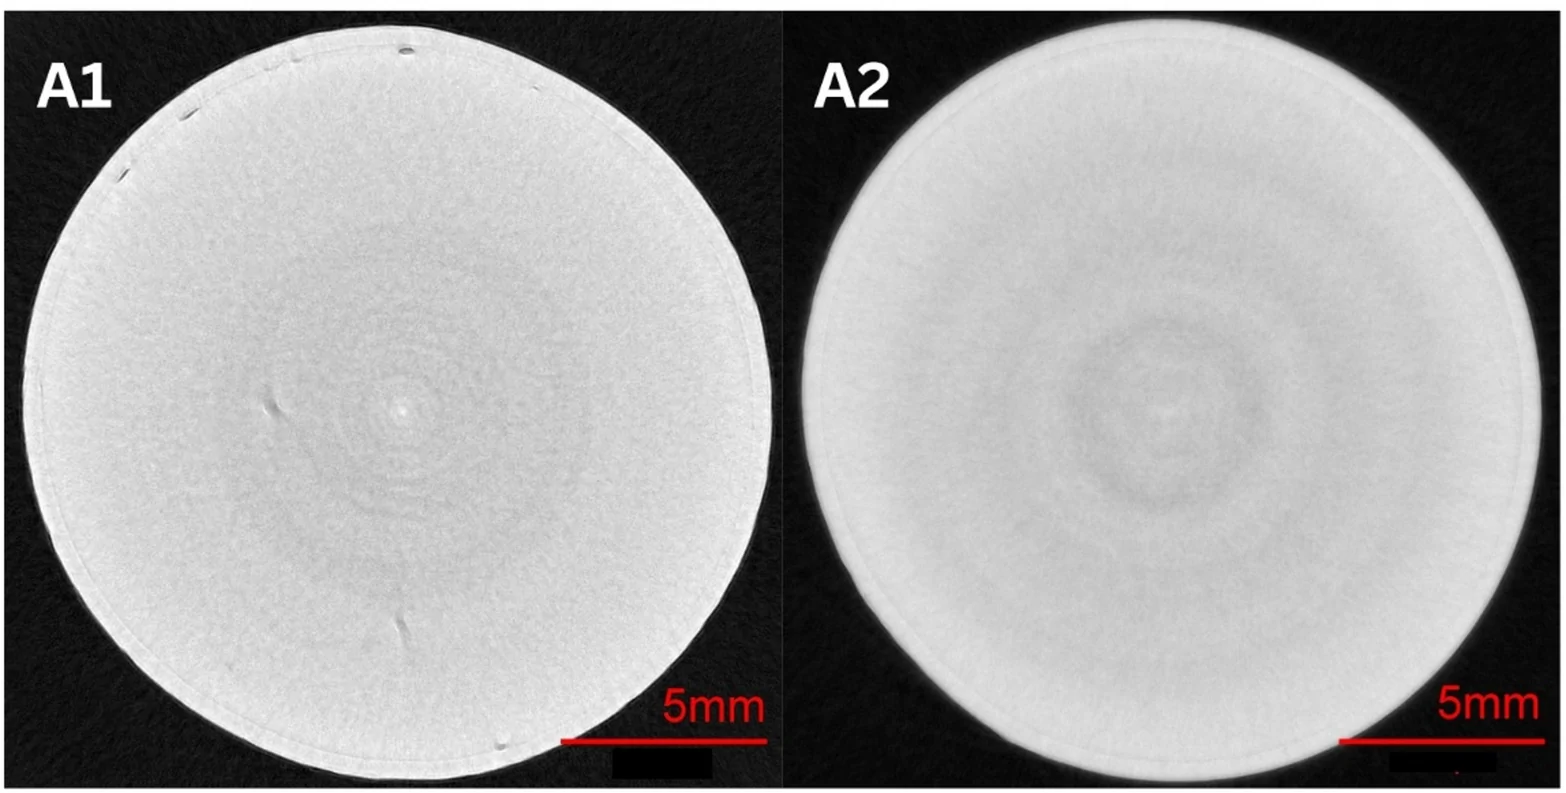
Axial CT slices of a sample container containing A1. Fresh SDFT tendon submerged in phosphate buffered saline (PBS), or A2. Fresh SDFT embedded in Low melting point agarose (LMPA).
